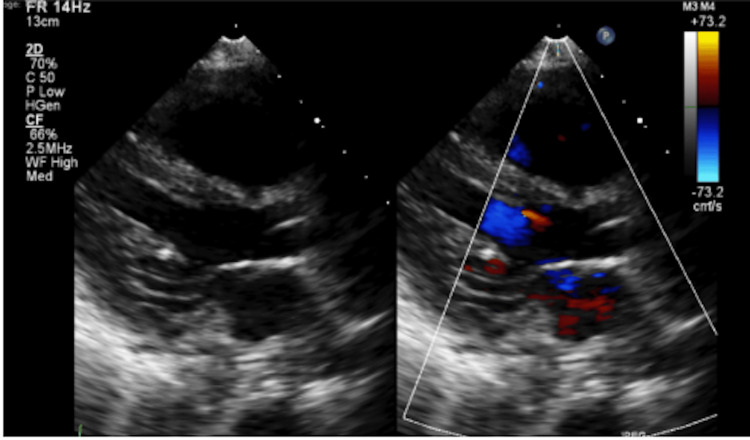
Bedside transthoracic echocardiography with color Doppler This image, performed during advanced respiratory failure, demonstrates cardiac function and flow patterns, providing a supportive hemodynamic assessment in the setting of multiorgan dysfunction and critical illness.
